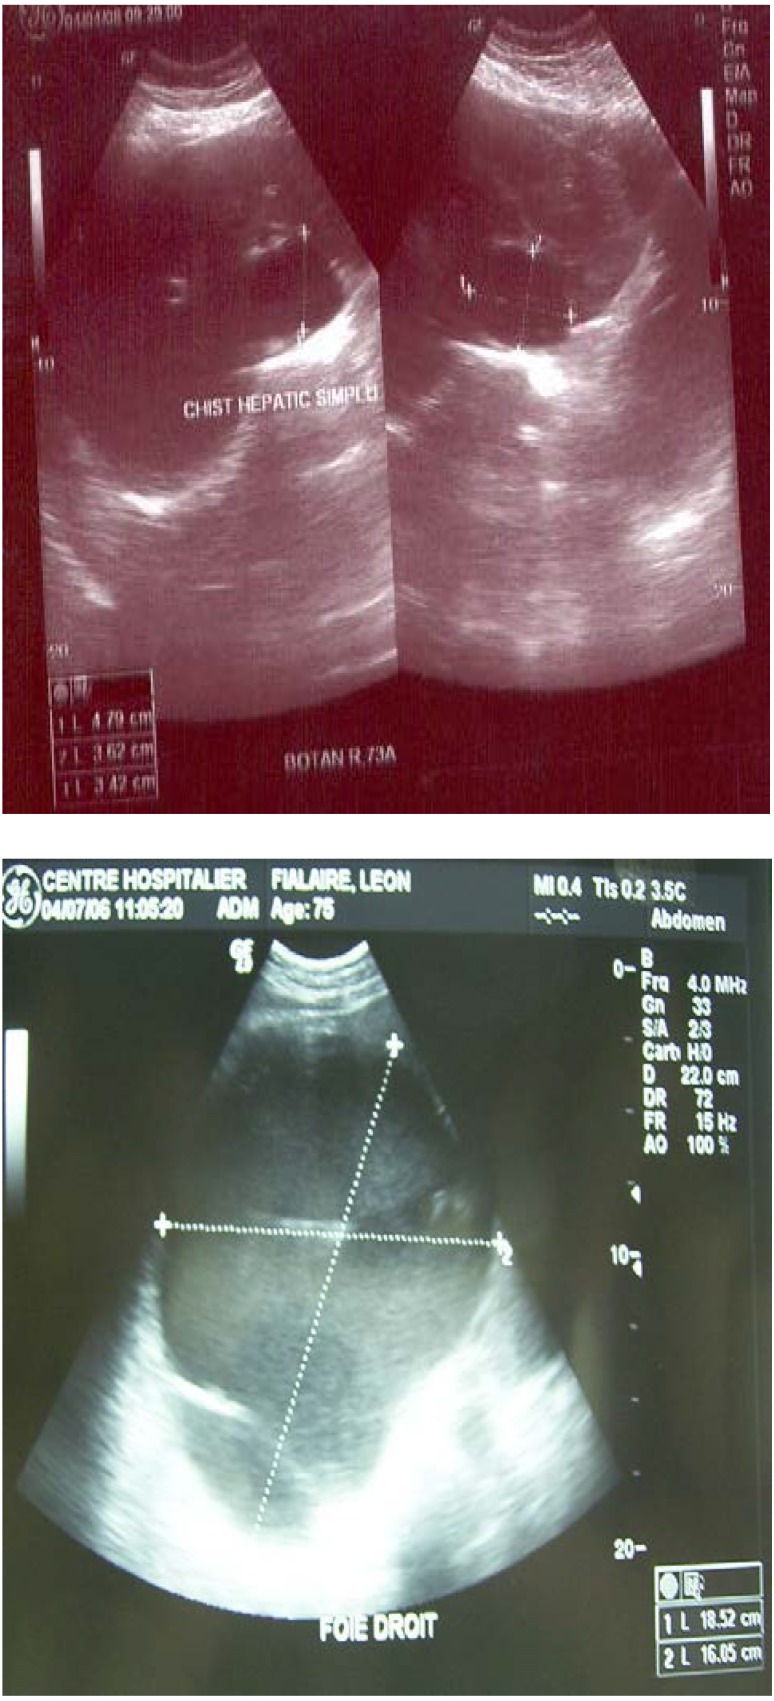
Ultrasound images from our study
